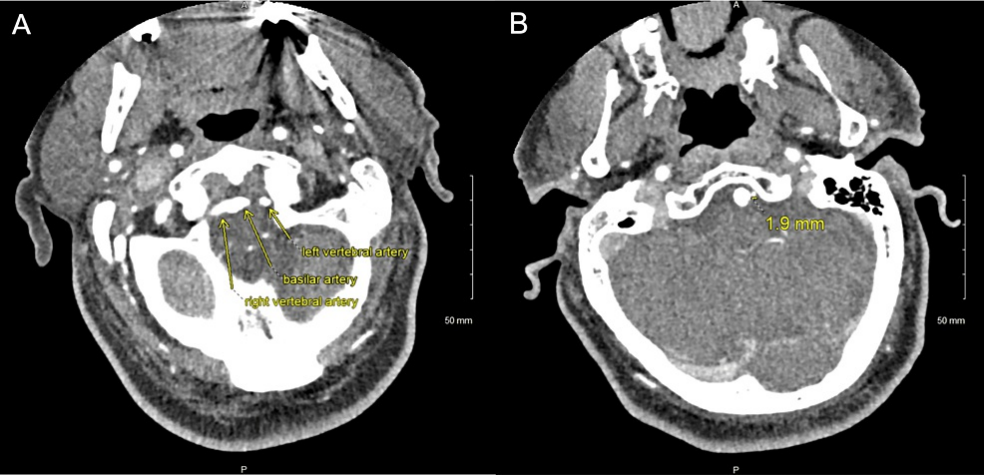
CT angiography of the head and neck
